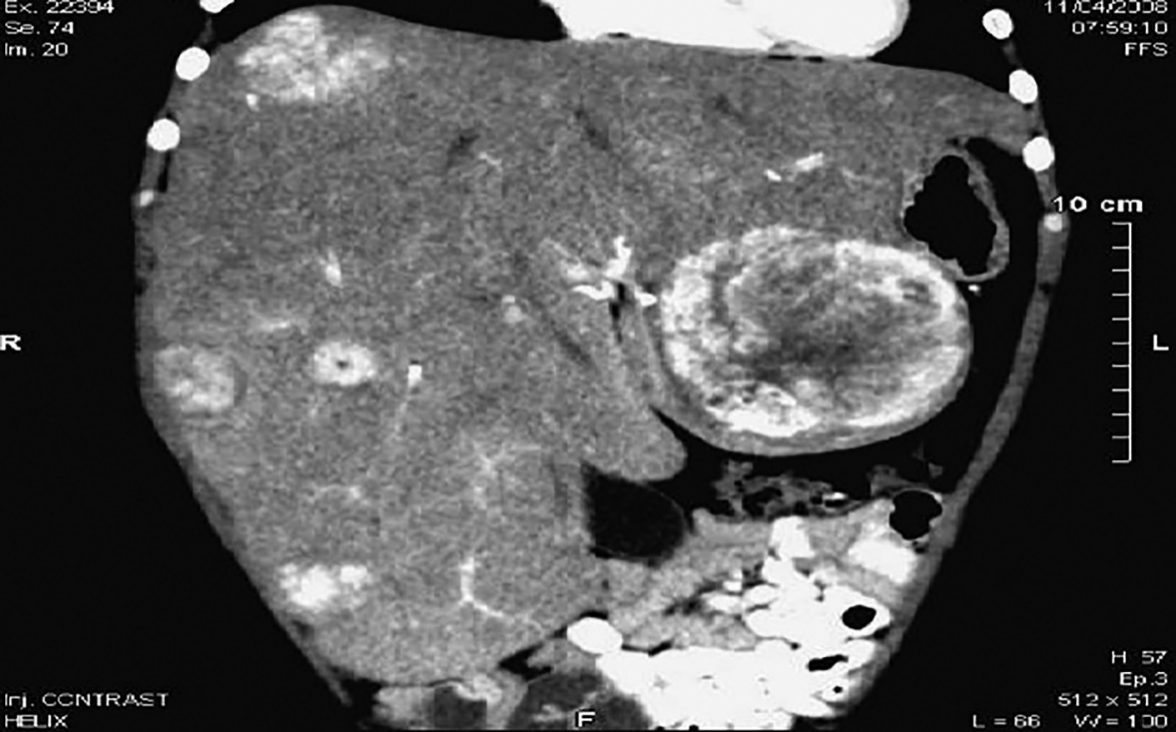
Liver MRI, multiple hepatic lesions, the largest of which is located in the left lobe of the liver and measures 10 cm, hyper T2 with heterogeneous enhancement after injection
